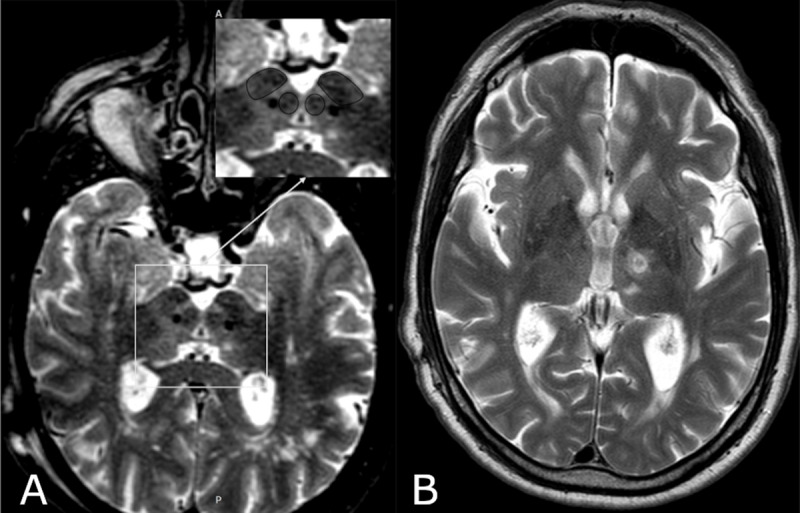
(a) Postoperative T2-weighted axial MRI demonstrating bilateral DBS lead placement in the posterior subthalamic area. The enlarged inset delineates anatomical boundaries of the subthalamic nucleus (STN) and red nucleus (RN). (b) Corresponding T2-weighted image showing the radiofrequency lesion in the left ventrolateral thalamus (Vim).
